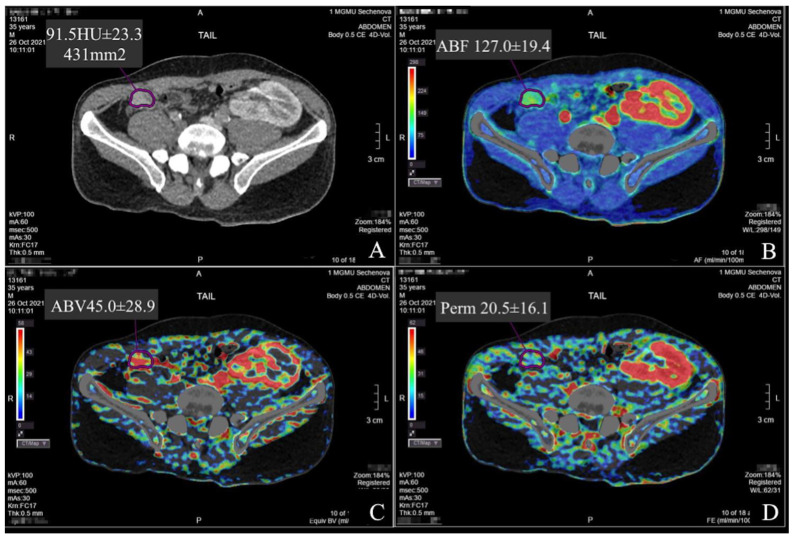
Volumetric blood flow indicators in the tail of the pancreas graft. (A) Conventional CT. The ROI is highlighted with a contour—pancreas graft; (B) arterial blood flow (ABF); (C) arterial blood volume (ABV); (D) permeability (Perm).
